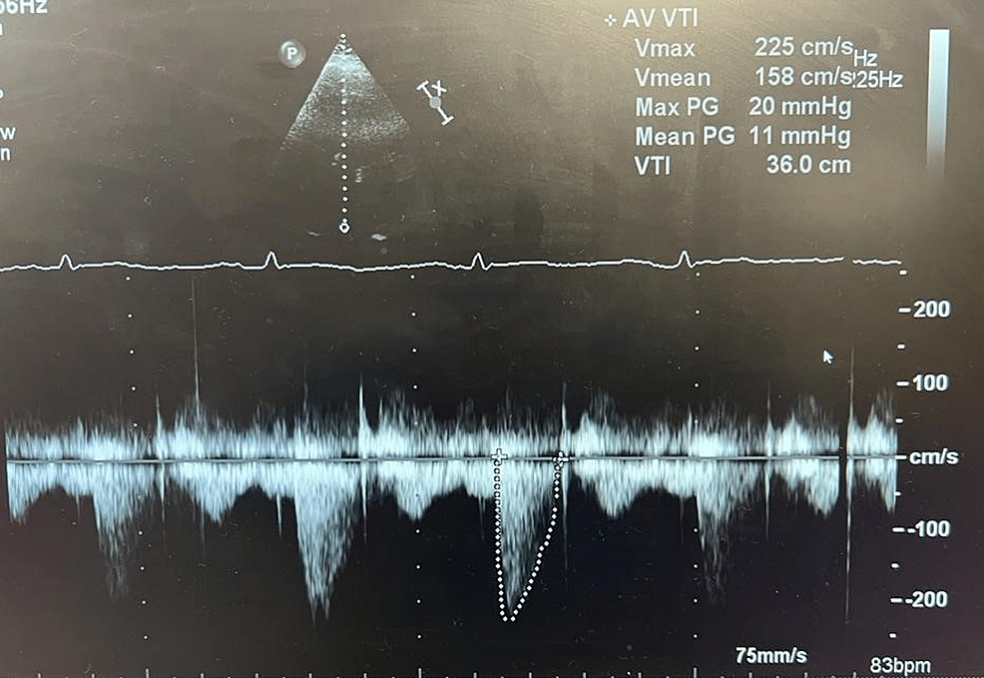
Aortic valve on echocardiography post-transcatheter aortic valve implantation, showing a mean aortic valve gradient of 11 mmHg and a maximum gradient of 20 mmHg
